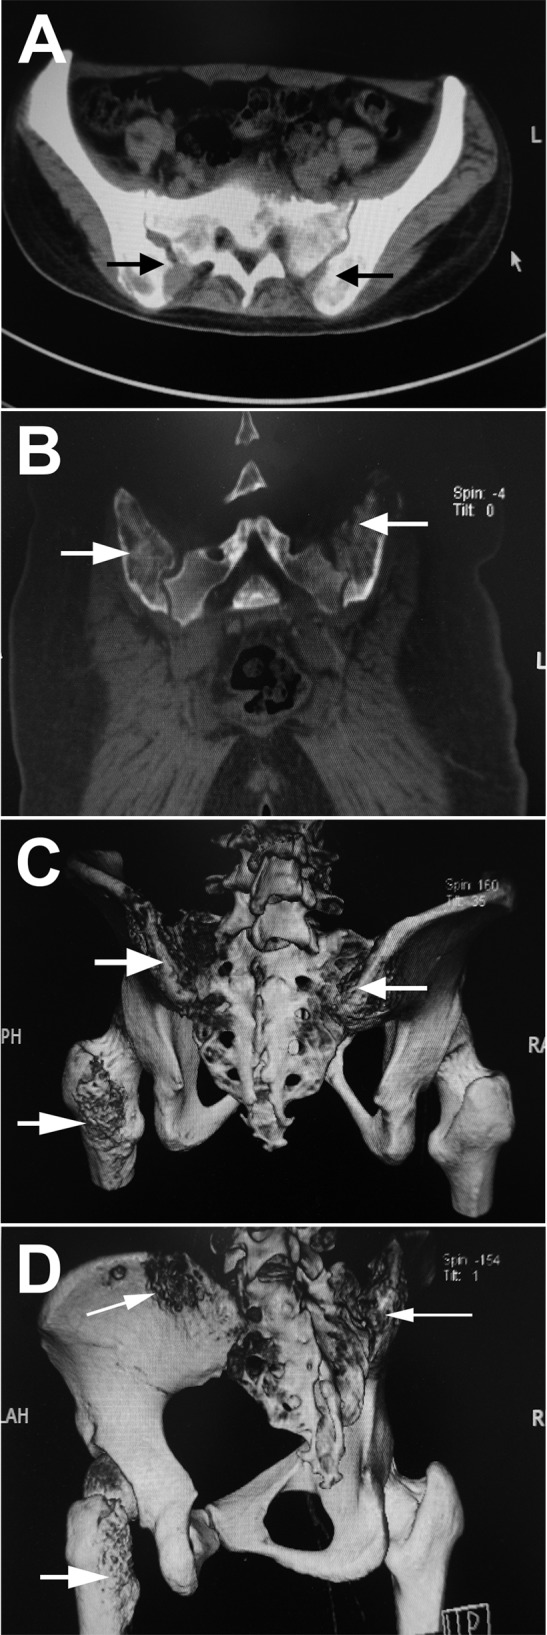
Computed tomography of the abdominal region and pelvis revealing severe osteolysis (arrows) around each sacroiliac joint and the left greater trochanter. However, no extraosseous involvement was visible, such as in the retroperitoneal and hepatic portal lymph nodes. (A) Horizontal position, (B) coronary position and (C and D) three-dimensional reconstructional views.
